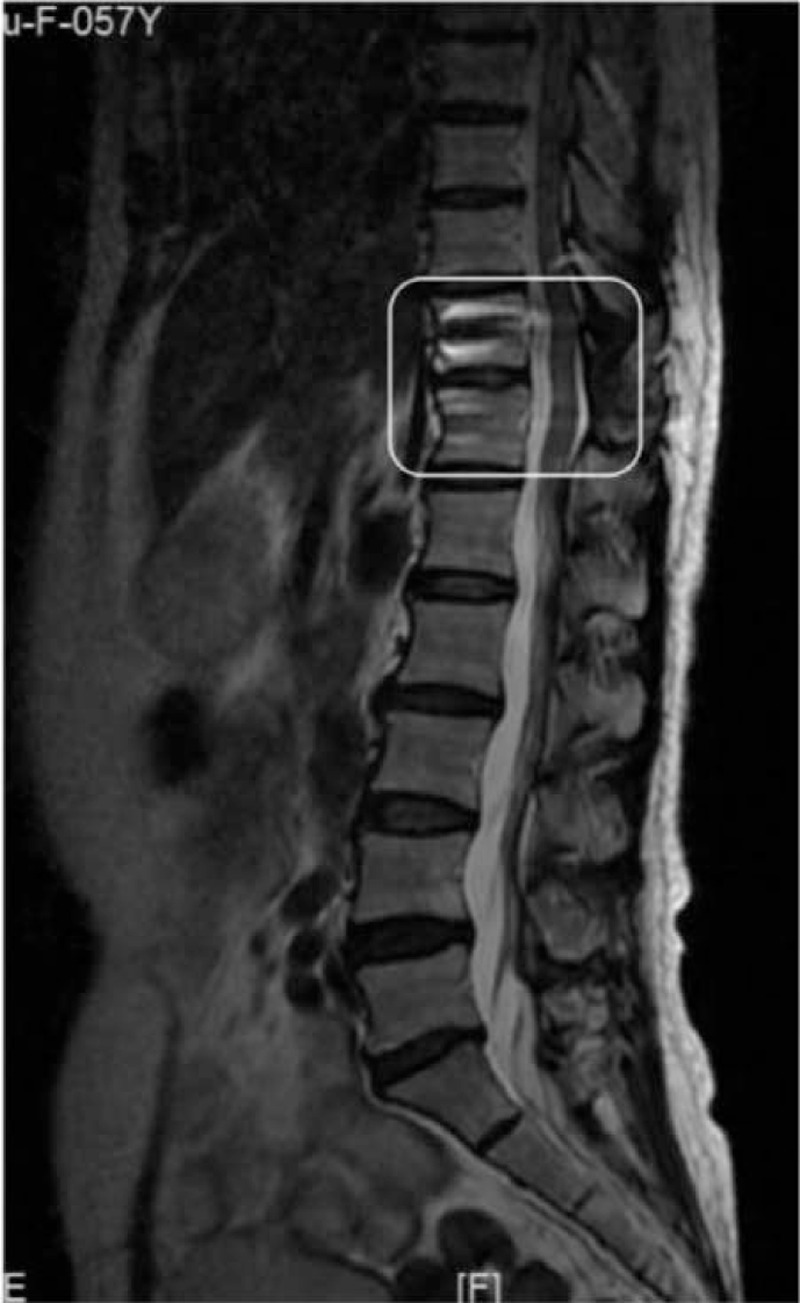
A sagittal T2-weighted image at 12-month follow-up shows an improvement in the high signal intensity on the T2-weighted image.
